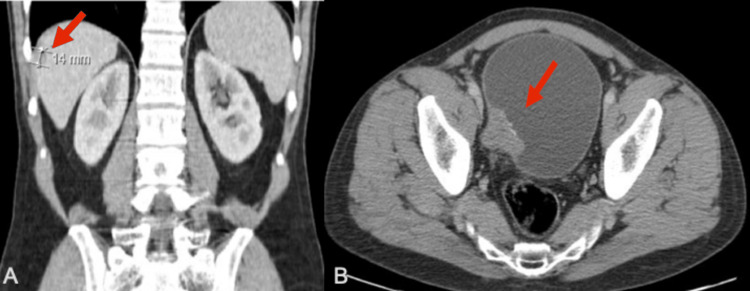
Imaging findings at presentation. A: CT scan showing a single liver metastasis (red arrow). B: CT scan showing an exophytic lesion on the right posterolateral wall of the bladder (red arrow). CT = computed tomography
